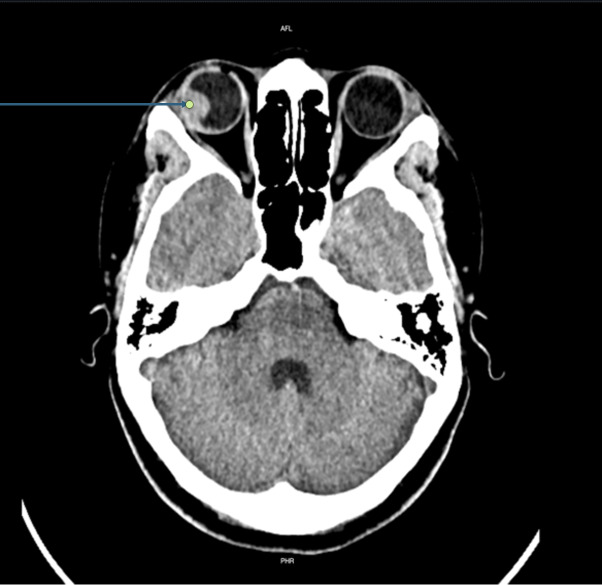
Non-contrast head computed tomography scan. The blue arrow indicates a space-occupying lesion in the right globe.
